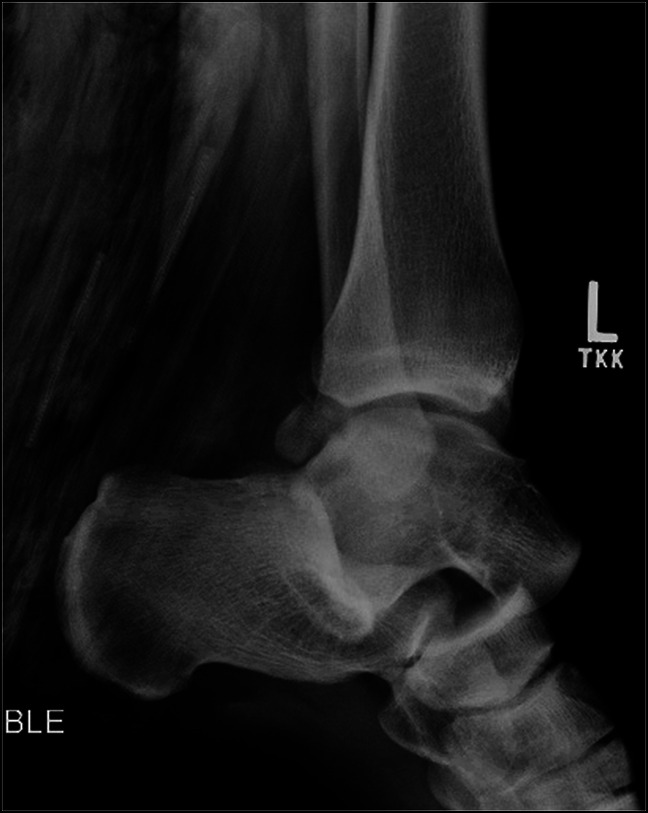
Prereduction lateral ankle radiograph showing anterior dislocation of the talus.
